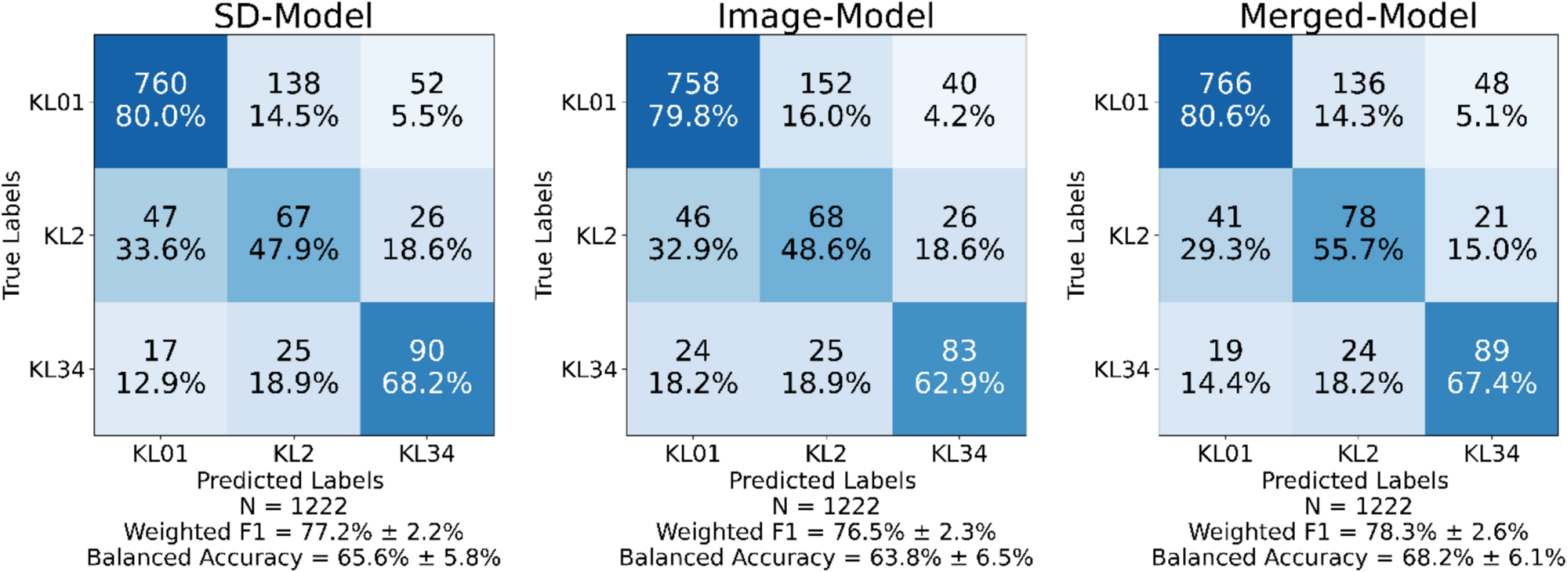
Confusion matrices for SD-Model trained on subject demographic (SD) predictors (1–3, 12 in Table 1), Image-Model trained on knee joint morphological predictors (4–12 in Table 1) from radiographs, and Merged-Model trained on a combination of demographic and knee joint morphological predictors (1–12 in Table 1)
